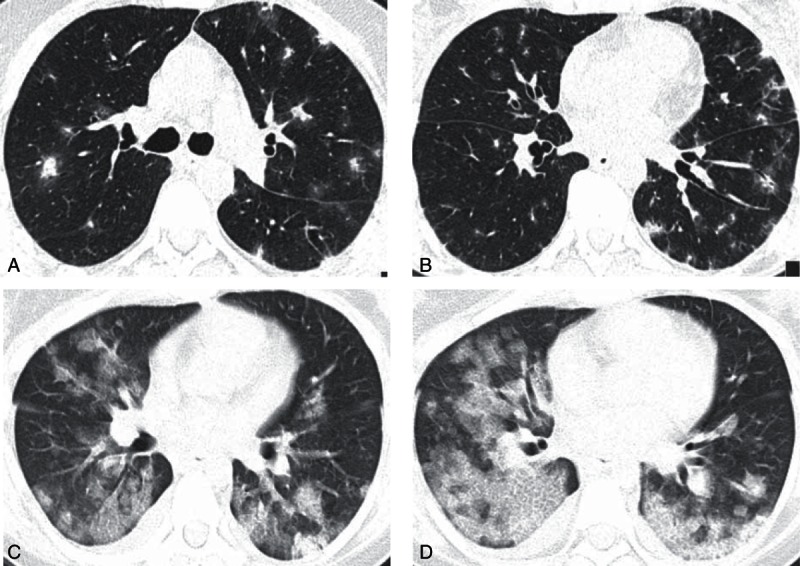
Radiological findings of acute fibrinous and organizing pneumonia. (A, B) High-resolution computed tomography images showing focal areas of airspace consolidation and ground glass along the bronchovascular bundles, with a diffuse random distribution. (C, D) Computed tomography images showing diffuse areas of airspace consolidation and ground glass along the bronchovascular bundles.
